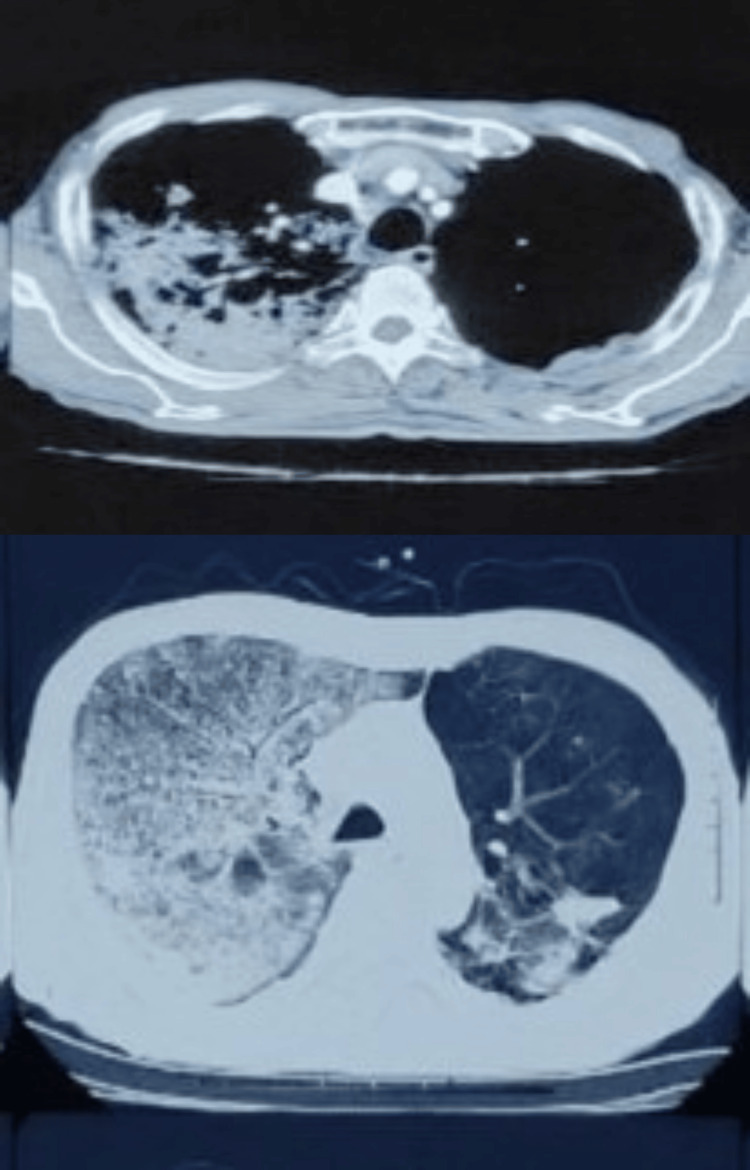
CT showing consolidation and ground glass opacities of the right upper lobe and multiple areas of air trapping seen in the left lung, along with a few fibrotic bands CT: computed tomography
